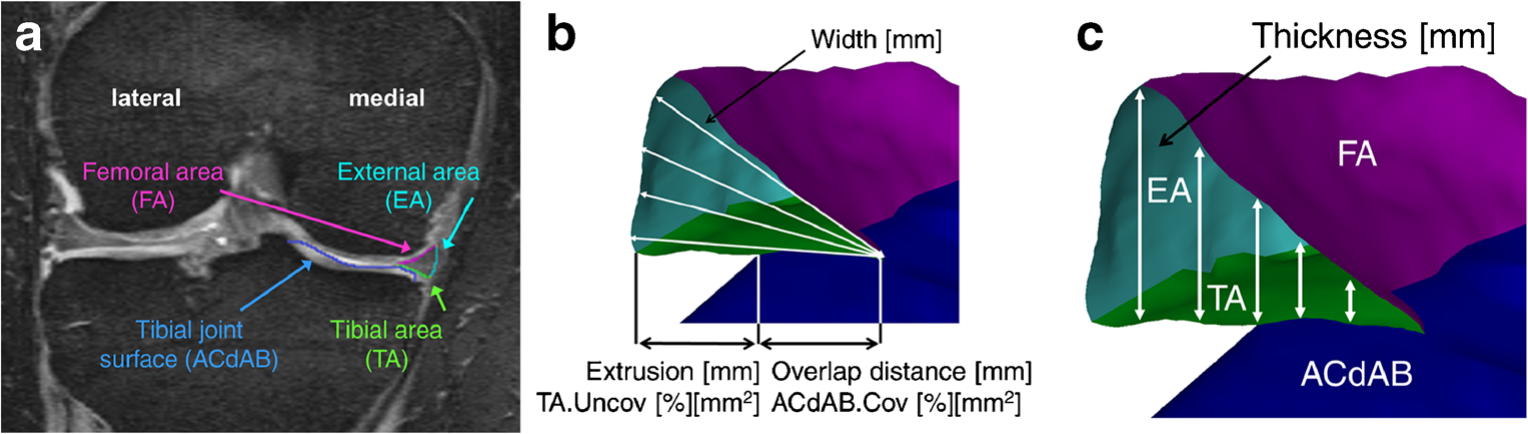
3D analysis of the menisci. MR imaging based, quantitative 3D analysis of the menisci in the medial compartment: a coronal MR image from a double echo steady state sequence (with water excitation) with segmentation of the medial meniscus; b–c 3D reconstruction of the medial meniscus depicting: ACdAB (joint surface of the tibia, consisting of the area of the cartilage surface, and denuded areas of subchondral bone if applicable); the femoral (FA), tibial (TA), and external surface (EA) of the medial meniscus; meniscal thickness and width; overlap area between TA and ACdAB (ACdAB.Cov), uncovered area of the TA (TA.Uncov), overlap distance (OvD) and extrusion distance (Ex)
